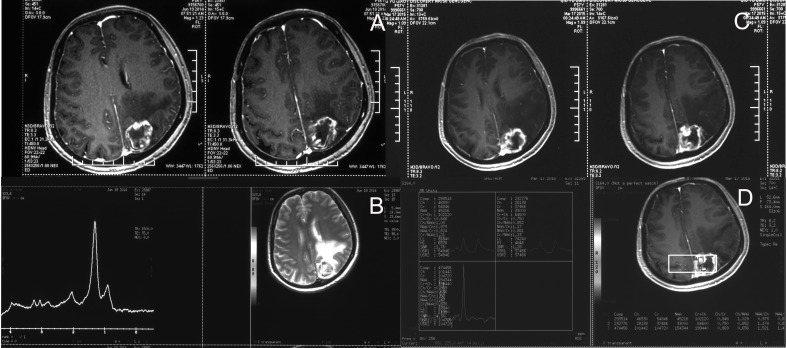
Brain necrosis progression and ineffective bevacizumab re-treatment A. In June 2014, enhanced MRI showed progression of the radiation brain necrosis; B. In June 2014, MRS indicated radiation brain necrosis(LL: 114334, Cho:20567, Cr:20432, NAA:38623), and the patient received 7 cycles of bevacizumab re-treatment from August 2014 to March 2015. C. In March 2015, enhanced MRI showed a more pronounced enhancement of the lesion than before and indicated treatment for brain necrosis was ineffective. D. In March 2015, MRS indicated brain necrosis (Cho:28138, Cr:37466, NAA:33000).
